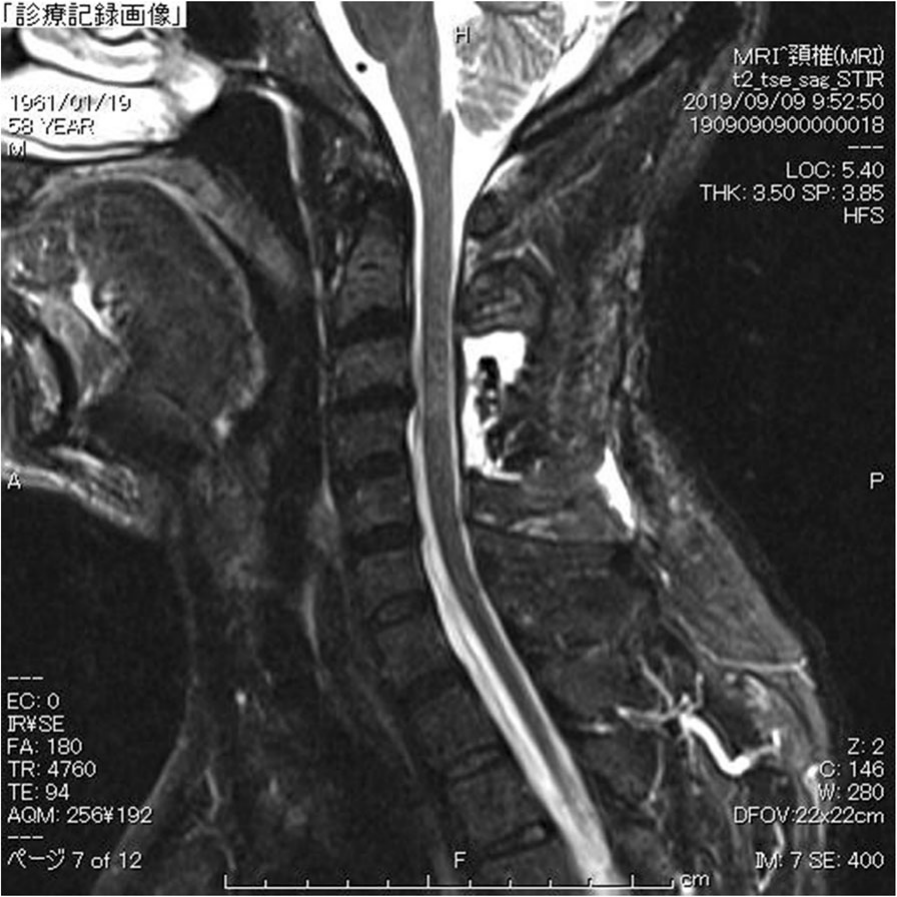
Postoperative imaging. Postoperative saggital view of T2-weighted fat-suppressed magnetic resonance imaging (MRI) in the cervical level revealed disappearing of the fluid collection in the retrospinal region at C1-C2 level. Also, the spinal canal stenosis at C3/4 level was treated
